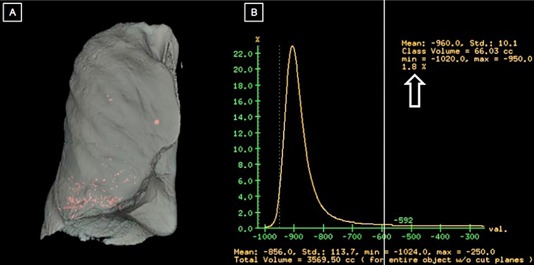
CT densitovolumetry: (a) 3D volume rendered CT shows the right total lung volume in white and the emphysematous volumes in red; (b) histogram of lung densities shows the emphysema index (large white arrow) and total lung volume.
